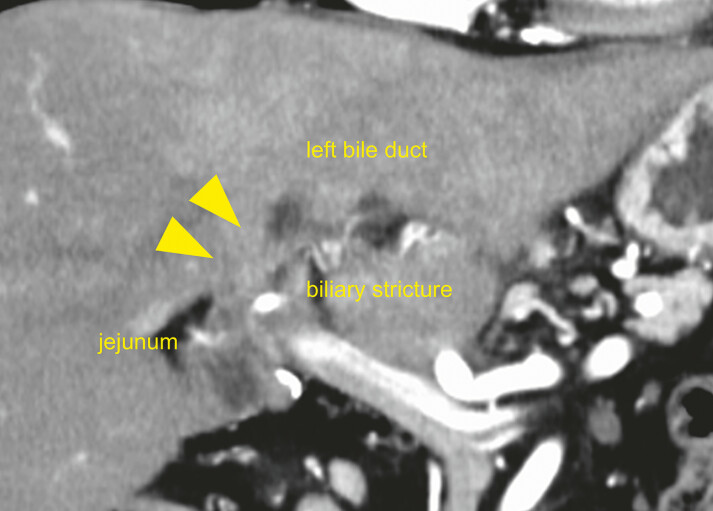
A contrast-enhanced CT image demonstrating a biliary stricture at the hepaticojejunostomy site (arrowheads), with upstream dilatation of the left bile duct. CT, computed tomography.
